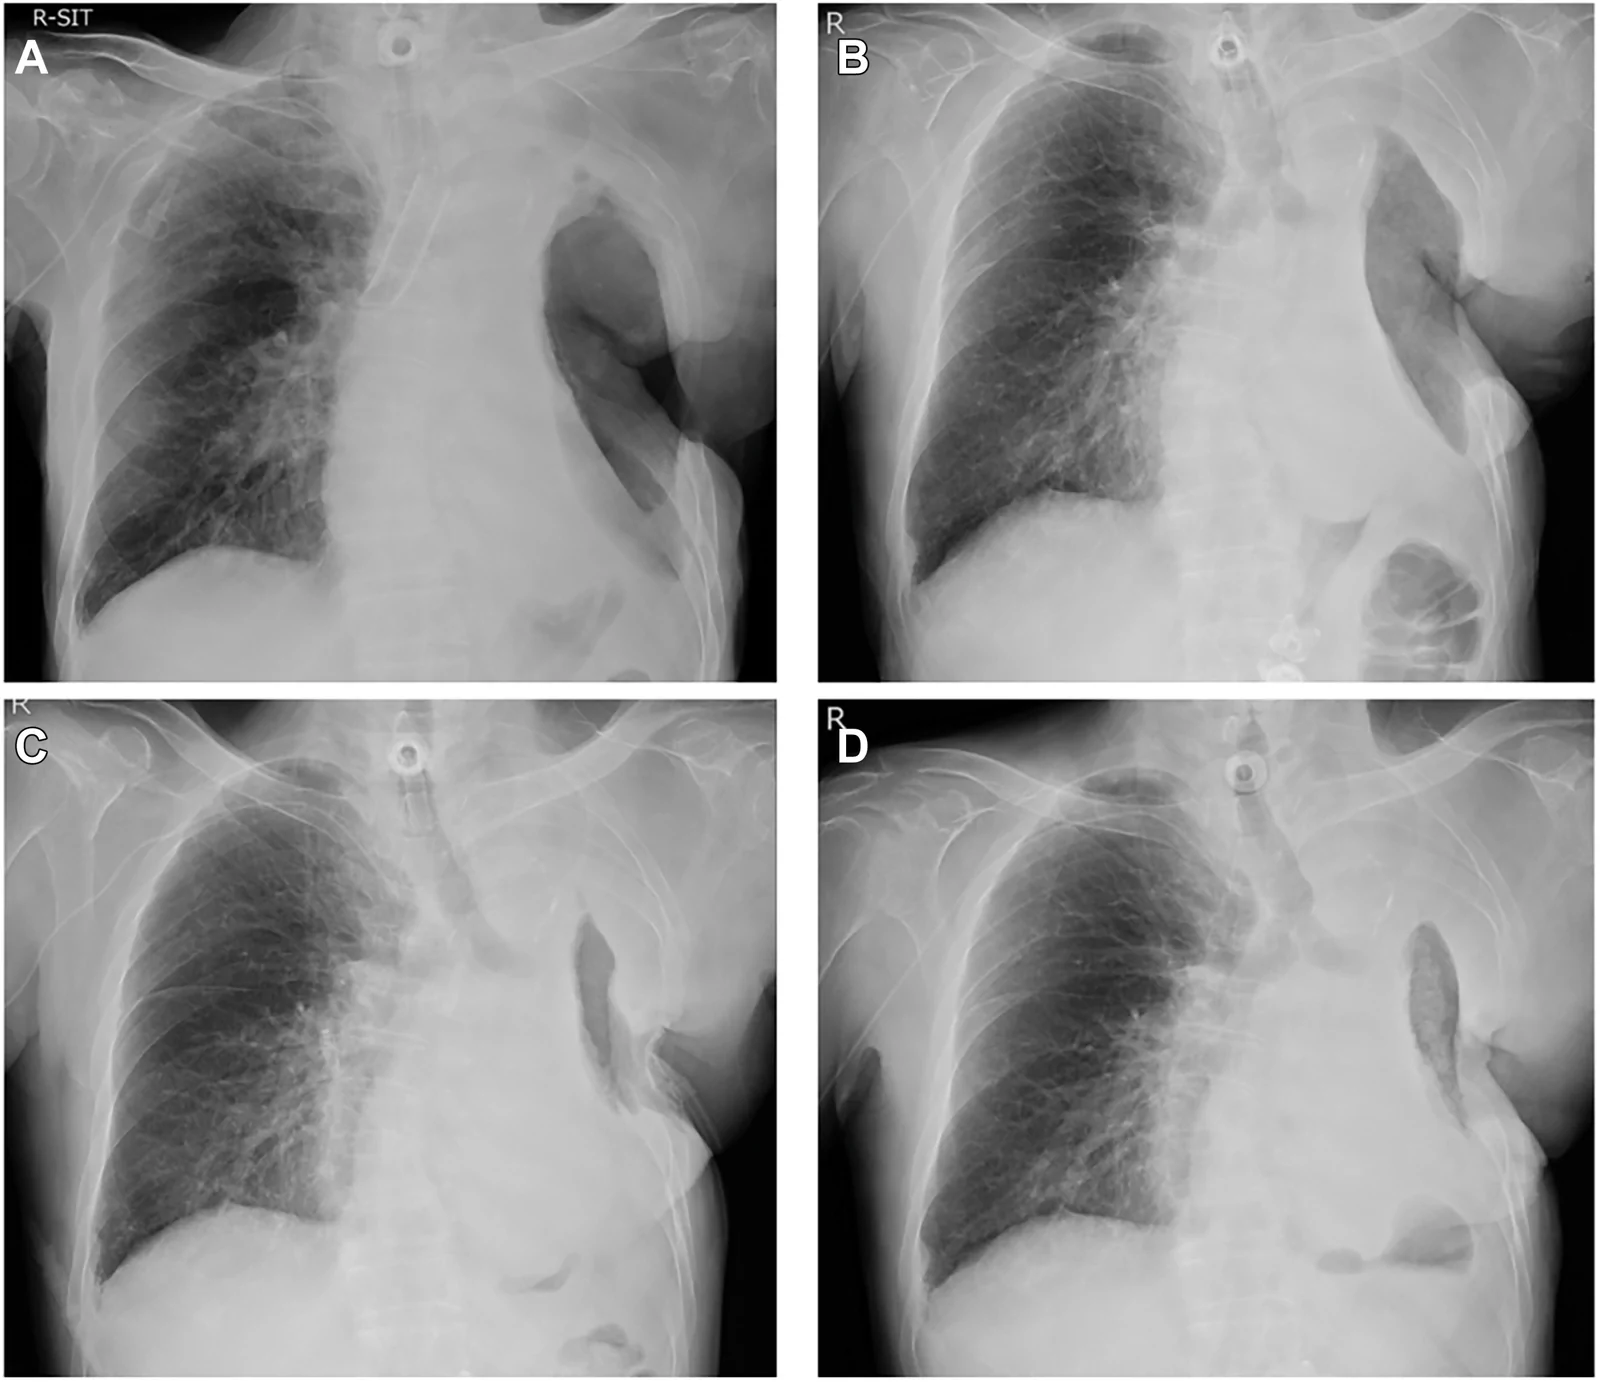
Serial chest radiographs showing progressive postpneumonectomy cavity reduction during negative-pressure wound therapy (NPWT). (A) At the time of fistula closure. (B) Two months later, pre-NPWT initiation, with only slight residual cavity reduction. (C) Day 4 of NPWT, with evident mediastinal shift and diaphragmatic elevation. (D) After NPWT completion, with residual cavity reduction <50% its original volume.
